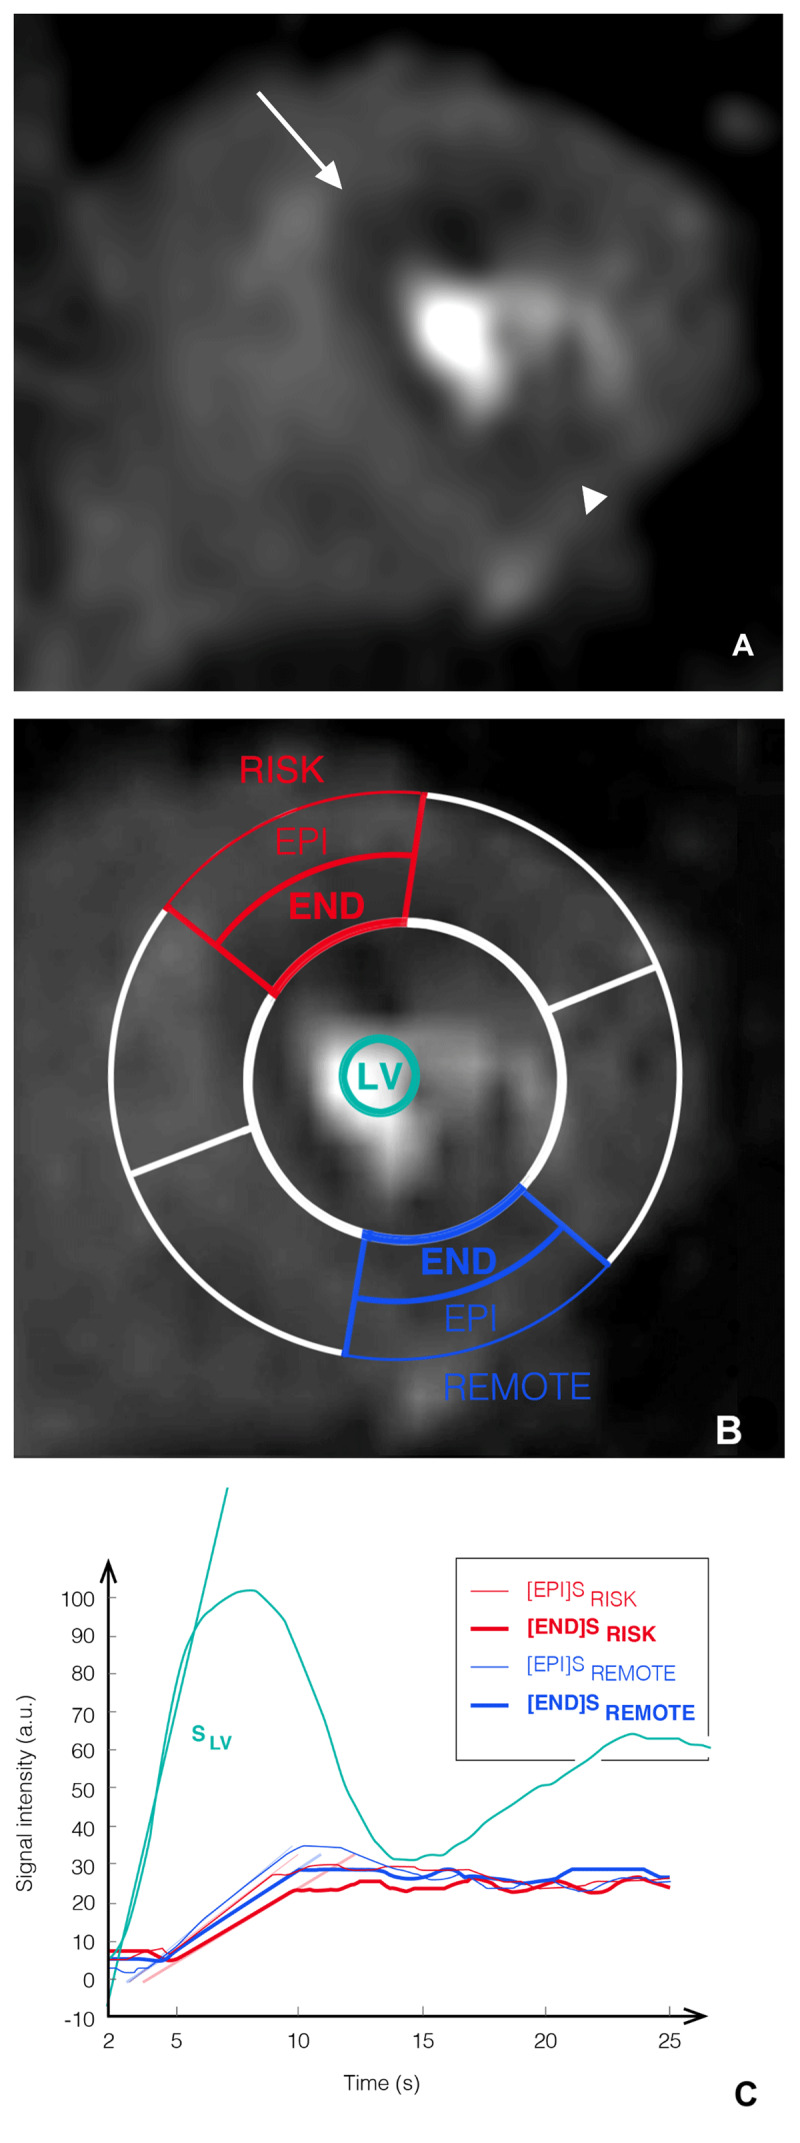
Semi-quantitative myocardial perfusion magnetic resonance imaging analysis. Detailed steps for semi-quantitative perfusion magnetic resonance imaging (MRI) analysis in a 69-year-old patient with intermediate-grade stenosis of the mid portion of the left anterior descending artery on both coronary computed tomography angiography and catheter coronary angiography (not shown). Peak myocardial enhancement on adenosine perfusion MRI showed a septal and anterior wall mid-left ventricular area of low signal intensity (white arrow, segment 7 in Figure 1A) in the area-at-risk (RISK), whereas the remote area (REMOTE) (arrowhead, segment 10 in Figure 1A) was homogenously enhanced. No abnormal enhancement was present on late-enhancement imaging (not shown). Equally divided subendocardial (bold lines, END) and subepicardial (thin lines, EPI) regions of interest are drawn in the RISK (red color) and REMOTE segments (blue color) after outlining the endocardial and epicardial borders of the myocardium during maximal hyperemia (Figure 1B). After extending these regions of interest to the whole frames, corresponding time-signal intensity curves and the maximal upslope value of the contrast enhancement were obtained (Figures 1C). RISK = myocardium beyond stenosis; REMOTE = remote myocardium; [END] = subendocardial; [EPI] = subepicardial; S= adenosine stress imaging.
